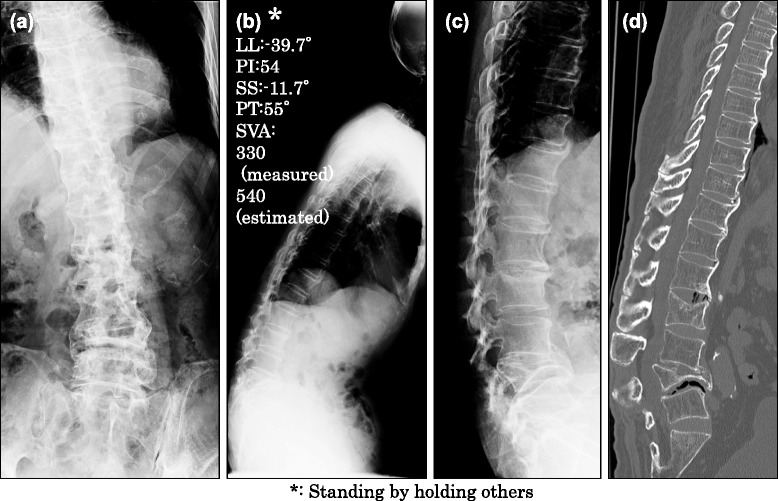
Plain radiograph showed rigid kyphoscoliosis. (a) The antero-posterior view showed slight left-convex scoliosis with deformity. (b) Whole spinal lateral view. Note that the patient was only able to maintain the standing position with assistance; thus, the image is not a true whole-spinal image. (c) The lateral view at maximum extension hardly showed changes in alignment. (d) Computed tomography scan sagittal image. LL: lumbar lordosis, PI: pelvic incidence, SS: sacral slope, PT: pelvic tilt, SVA: sagittal vertical axis.
